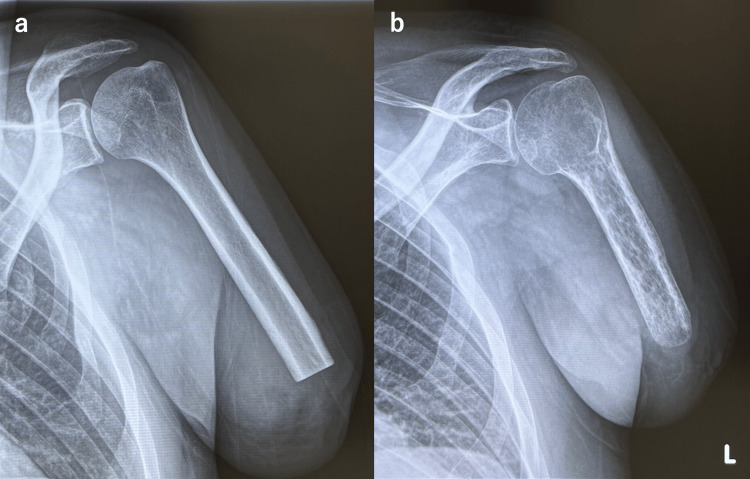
Plain radiographs of the stump in Case 1. (a) One month after surgery. The humeral length is 18.8 cm. (b) Nine months after surgery. The humeral length is 15.3 cm. The humeral length was measured along a straight line from the greater tubercle to the distal end of the humerus.
